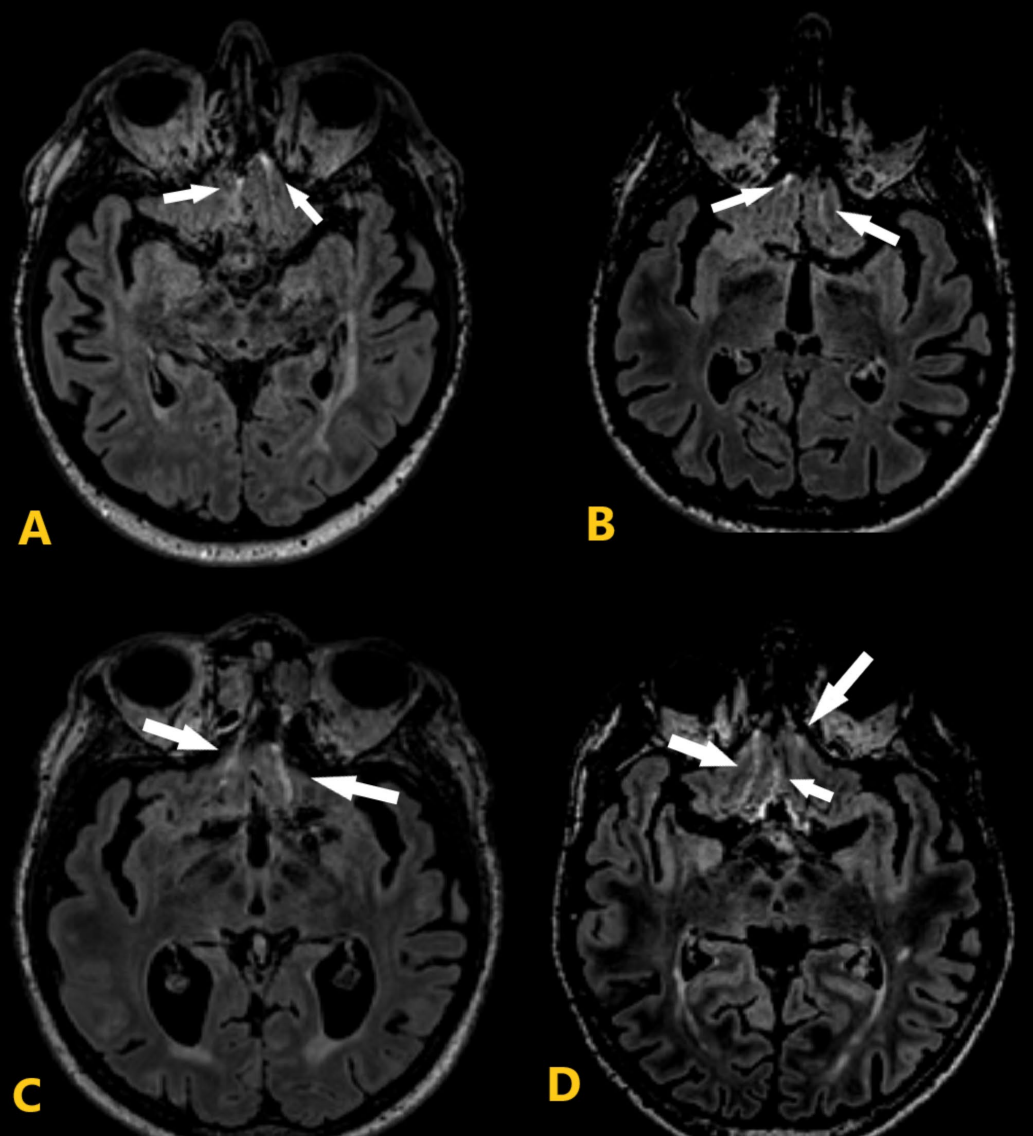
Example of Inferior Frontal Sulcal Hyperintensity (IFSH) on fluid-attenuated inversion recovery (FLAIR) images. The IFSH score in each of the three inferior frontal sulci was assessed above the reference slice. (A) The IFSH total score was 2 (see white arrows: one point for the central sulcus and one point for the left sulcus). (B) The IFSH total score was 3 (1 point for the right sulcus and two points for the left sulcus). (C) The IFSH total score was 5 (two points for the right sulcus and three points for the left sulcus). (D) The total IFSH score was 6 (three points for the right, two points for the central sulcus, and one point for the left sulcus.
